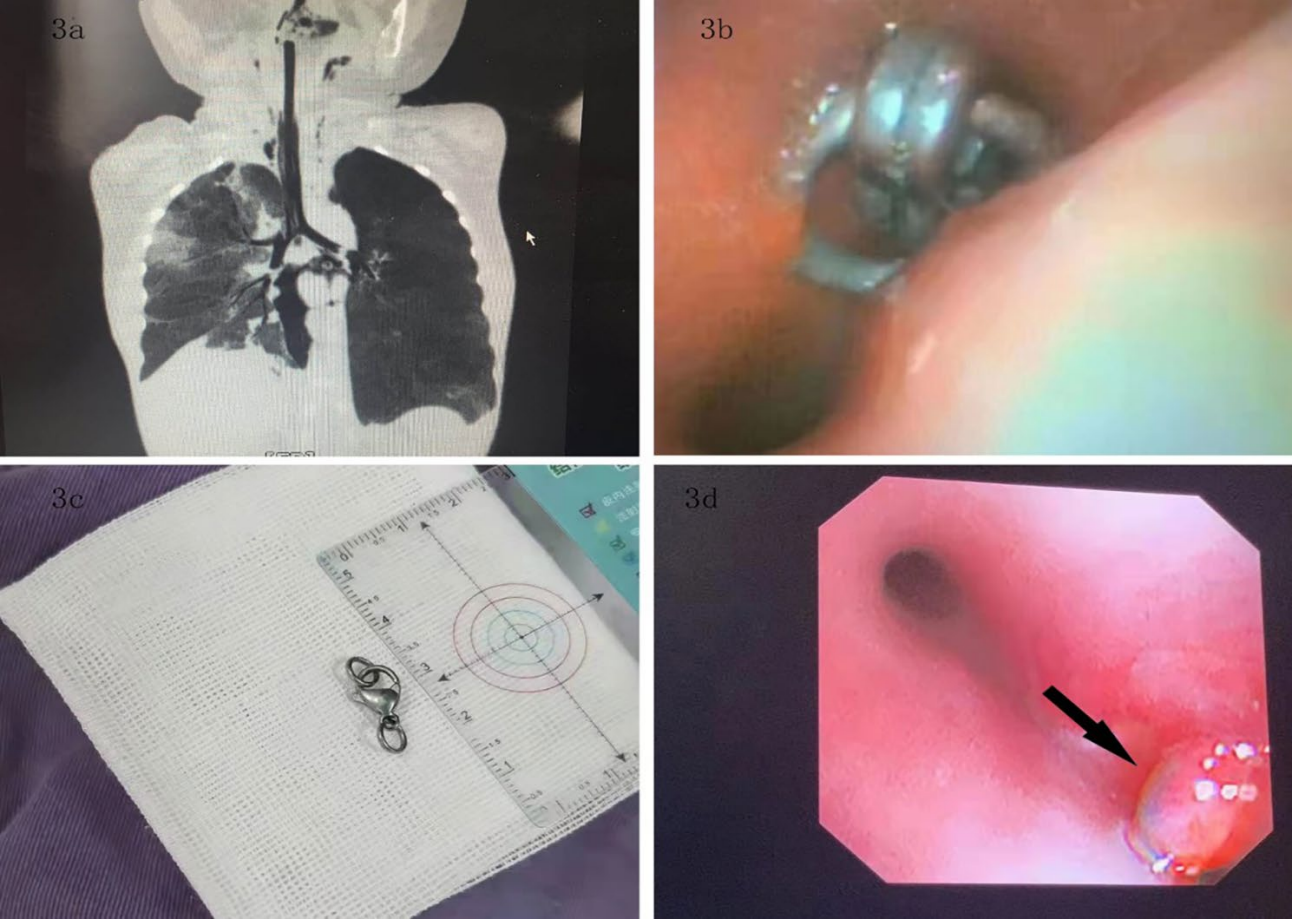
a: Emphysema and mediastinal emphysema on lung CT after choking on a solid foreign body; b: The tracheoscopic view of a metallic foreign body in the opening of the right main bronchus; c: The foreign object is a metal necklace clasp; d: Granulomatous hyperplasia of the right main bronchial opening visible after the removal of the foreign body
